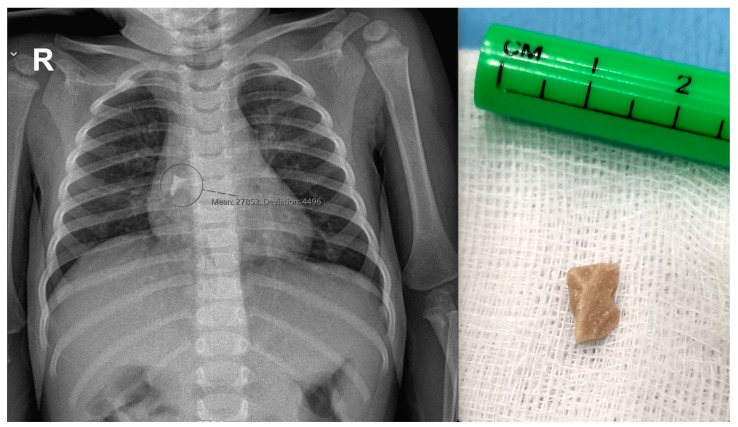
X-ray finding of a stone in the middle lobar bronchus, just below the branching of the right main bronchus of a 15-month-old girl (left), and the stone after successful removal with forceps using rigid bronchoscopy (right).
